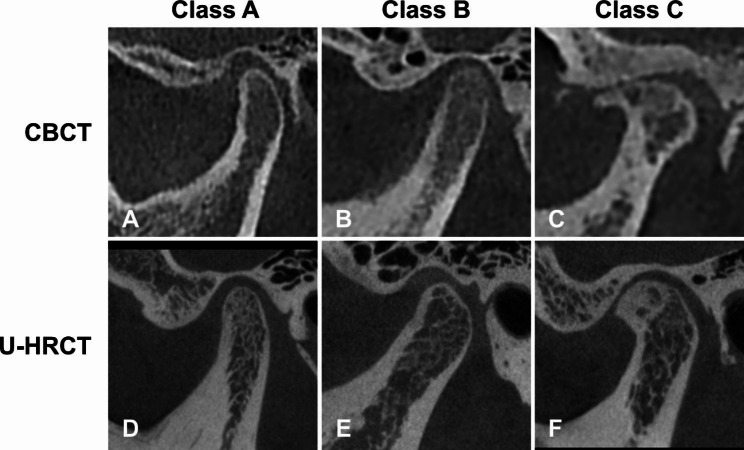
Diagnostic classification for TMD using CBCT and U-HRCT. Class A = no evident lesion (A and D), Class C = definitive lesion, including generalized subchondral sclerosis, osteophyte, erosion of the condyle, subchondral cyst and condylar flattening (C and F), and Class B = ambiguous condition where a case could not be diagnosed as Class A or C (B and E)
